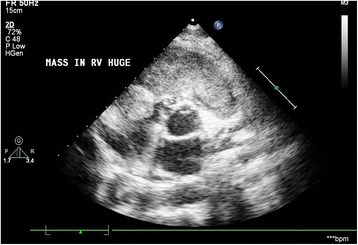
Preoperative echocardiogram showing right ventricular mass.
